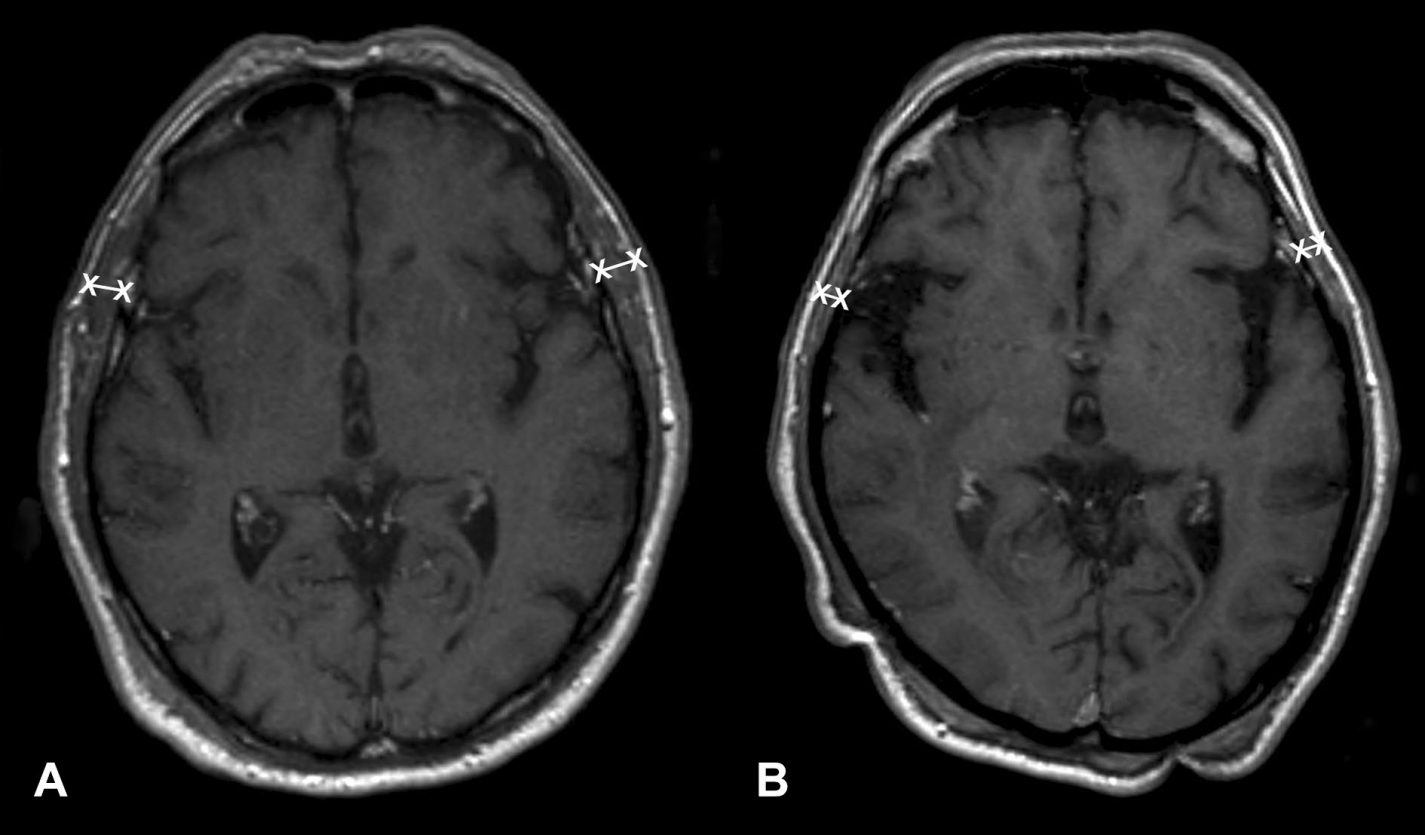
TMT assessment represented on brain MR images. a A 77-year-old male patient with an overall survival of 15 months (median TMT = 8.8 mm), and b a 73-year-old male patient with an overall survival of 3 month (median TMT = 2.8 mm)
